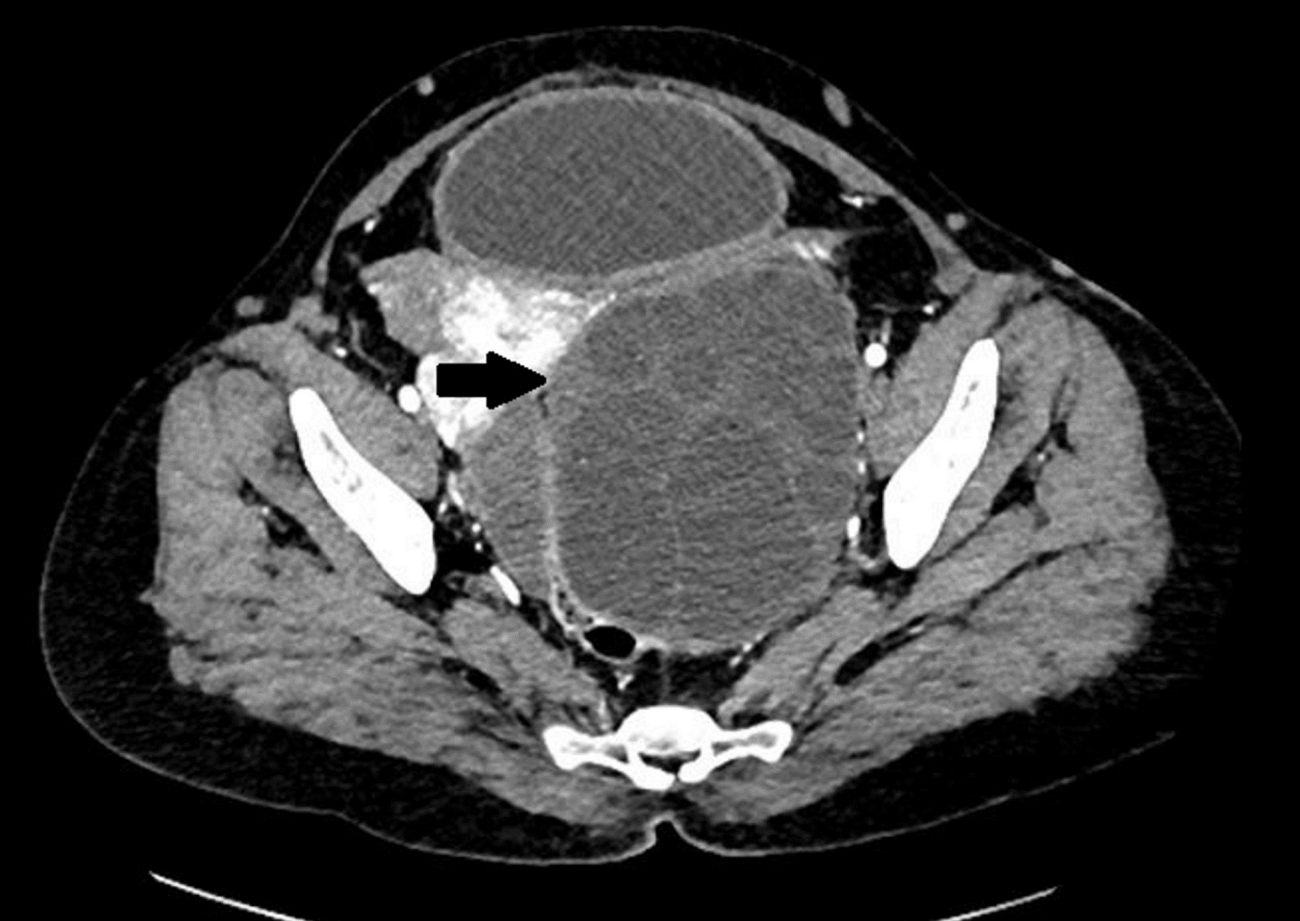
Adnexal hydatid cyst on CT CECT abdomen axial image shows a large, well-defined, rounded, thin-walled, multiloculated cystic lesion in the pelvic cavity on the left side. Left ovary is not visualized separately. CECT: contrast-enhanced computed tomography, CT: computed tomography
